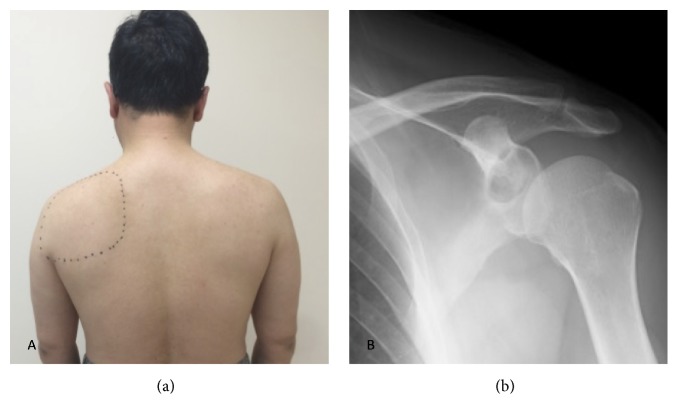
(a) Clinical photograph before the surgery shows a dotted circle at the left shoulder that indicates the area at which the patient complained of pain. The area includes the supra- and infraspinatus muscles and the suprascapular nerve. (b) Radiograph of the left shoulder shows a radiolucent cystic lesion in the superior glenoid.
